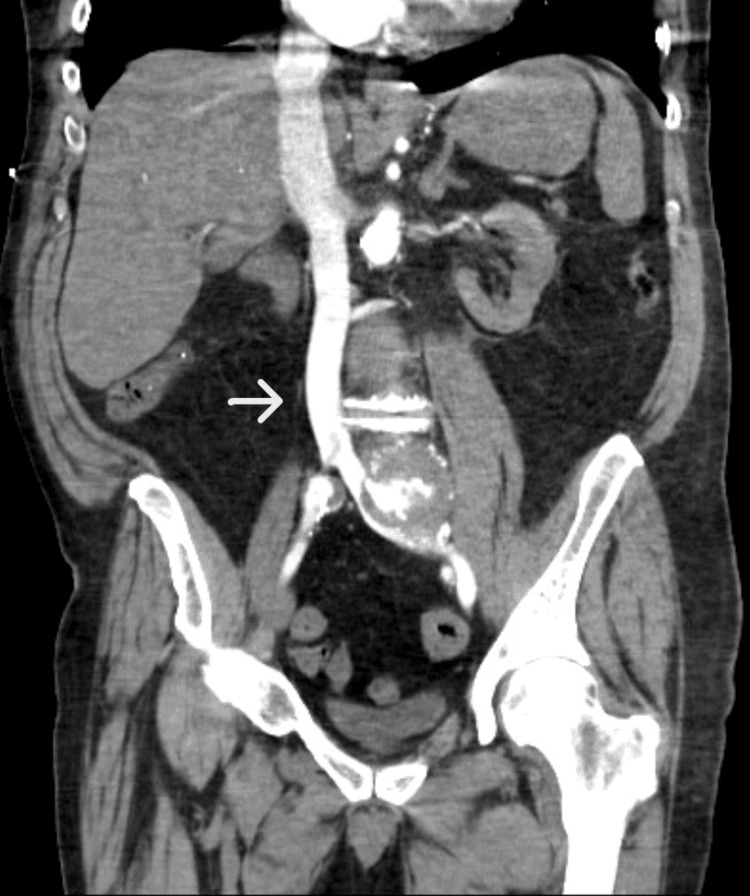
CT with IV contrast demonstrating reflux of contrast in the inferior vena cava secondary to fistula (arrow)
